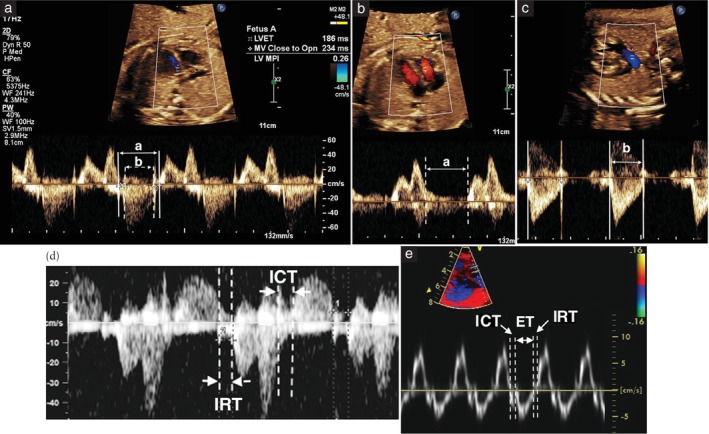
Assessment of myocardial performance index (MPI). (a) Left ventricular (LV) inflow‐outflow Doppler waveform with a sample gate placed within the LV in a location where both inflow (above baseline) and outflow (below baseline) signals are captured simultaneously, with measurement of ‘a’ (offset of one inflow to onset of next inflow) and ‘b’ (ejection time (ET)). Note the ‘valve clicks’, which can guide measurement. The MPI is calculated using the equation (a − b)/b. (b,c) Calculation of MPI for the right ventricle (RV) requires separate inflow and outflow Doppler signals. (b) From inflow tracings, ‘a’ can be measured from the offset of one inflow to the onset of the other. (c) From the outflow tracings, ‘b’ is measured as the ET. (d) The numerator in the MPI equation (a − b) equates to the sum of the isovolumetric relaxation time (IRT, from offset of outflow to onset of inflow) and isovolumetric contraction time (ICT, from offset of inflow to onset of outflow), as measured in this LV inflow‐outflow Doppler waveform. (e) MPI can also be assessed using tissue Doppler for both RV and LV. Similarly, valve clicks are helpful to guide measurement. Unlike with pulsed‐wave Doppler, a simultaneous inflow‐outflow tissue Doppler signal can be used to measure RV‐MPI. A major disadvantage is lack of availability of tissue Doppler imaging software/resources on many ultrasound systems.
